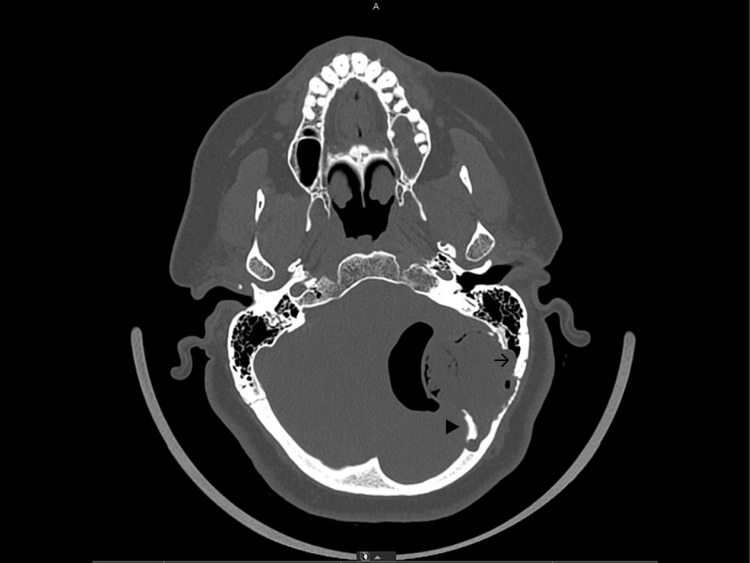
Preoperative CT scan showing erosion of the anteromedial part of the mastoid air cells (arrow) and inward displacement of the internal table (triangle).
